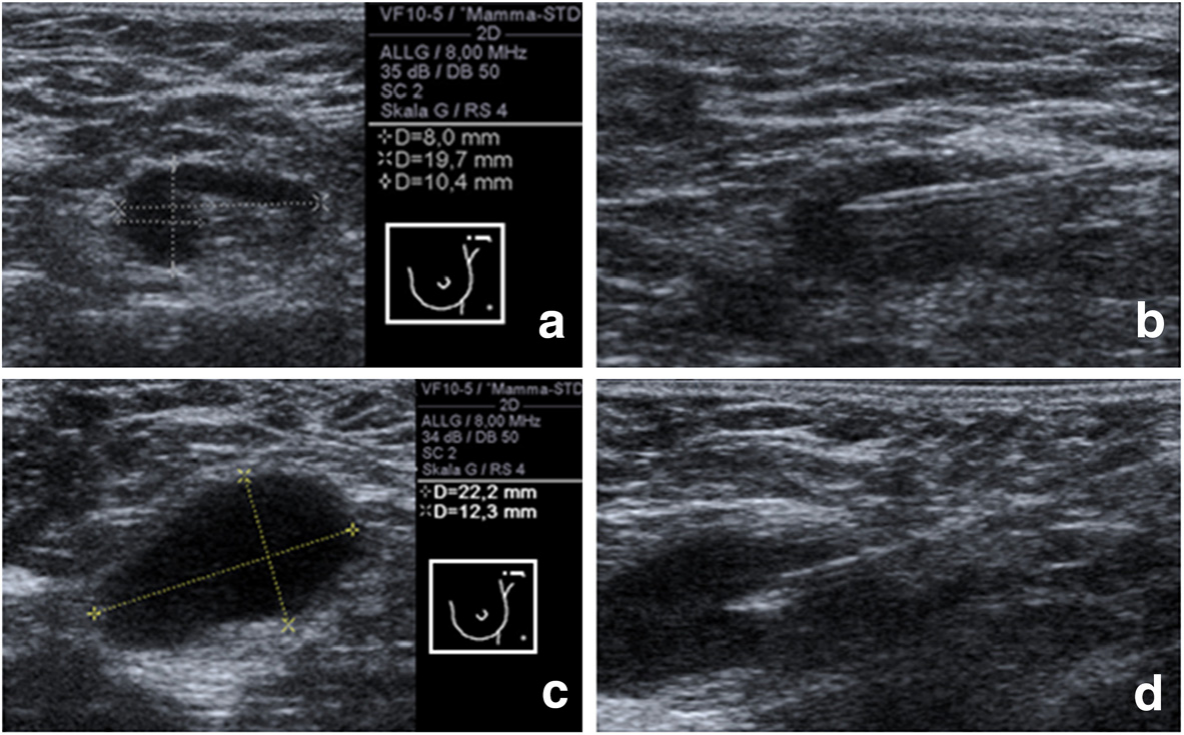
Examples of sonographically suspicious axillary lymph nodes that received further fine needle aspiration cytology and cytological evaluation. a Lymph node presenting a normal size, a longitudinal/transversal ratio < 2, a focal thickening of the cortex (8 mm) and an eccentric hilum. b Ultrasound-guided fine-needle aspiration cytology. c Lymph node with a round shape (longitudinal/transversal ratio < 2), an indeterminate size and loss of fatty hilum. d Ultrasound-guided fine-needle aspiration cytology
